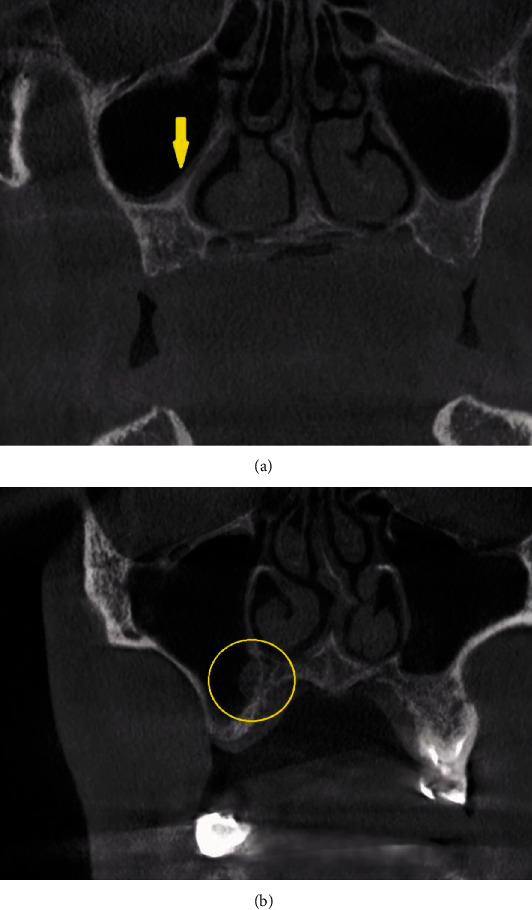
(a) Coronal CBCT section showing evidence of mucosal thickening (yellow arrow). (b) Coronal CBCT section showing mucous-retention cyst in the right maxillary sinus proximal to the osteotomy site (yellow circle).
